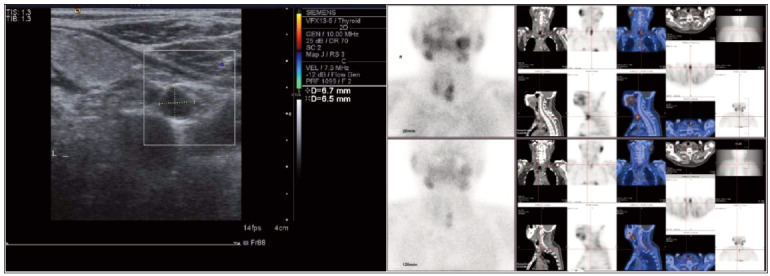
B-scan ultrasonography, ETC examination of secondary hyperparathyroidism.<br>Note: The results of B-scan ultrasonography on the left side suggested hypoechoic inferior lateral pole of the left thyroid; the ETC examination findings on the right side indicated a delayed uptake of MIBI lesion in the lower portion of the left lobe thyroid, prompting consideration of the parathyroid gland’s location and the potential for adenomatous hyperplasia; small nodules posterior to the middle of the right lobe thyroid.
